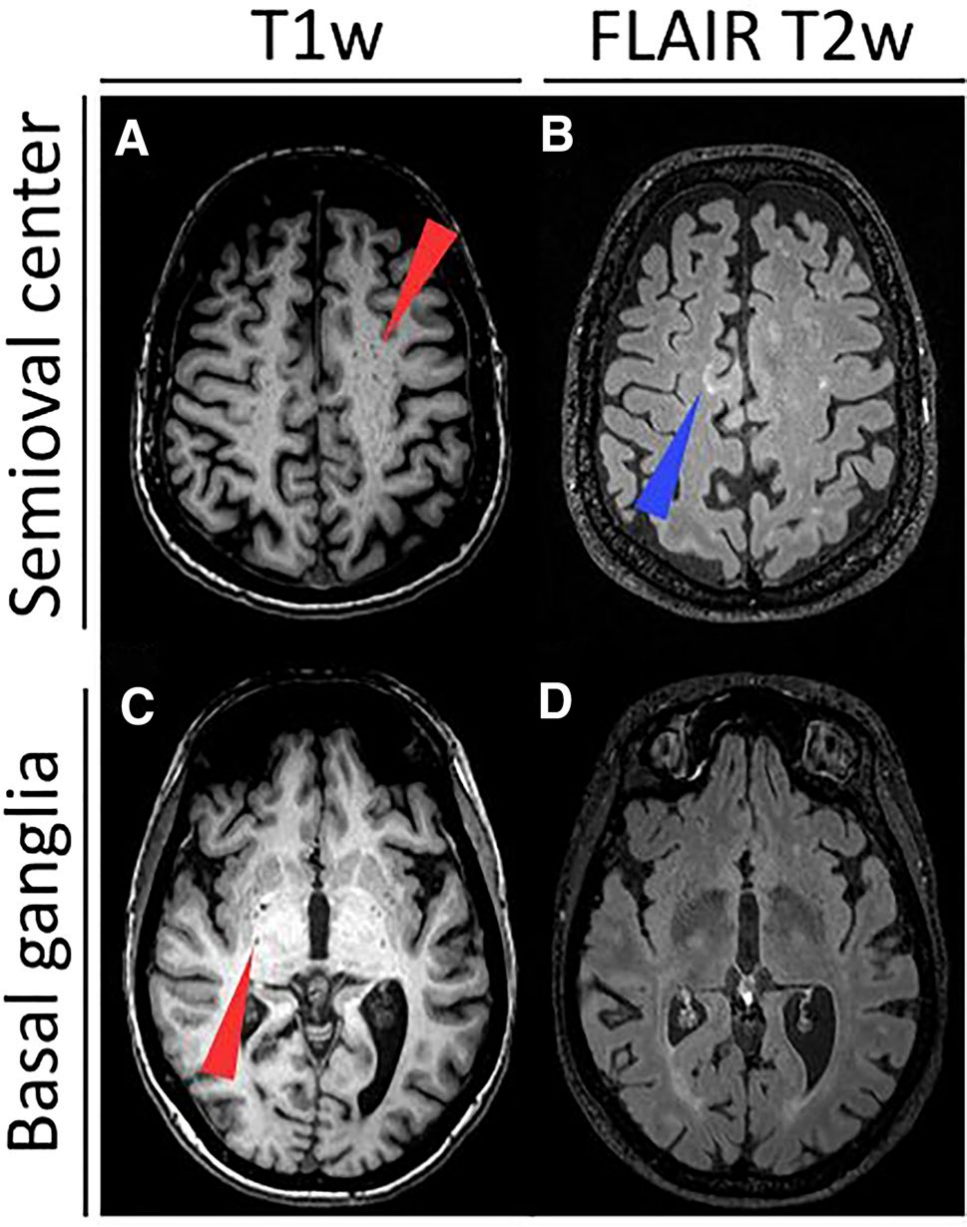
Enlarged perivascular spaces (EPVS) are readily detectable on T1- and fluid-attenuated inversion recovery (FLAIR) T2-weighted (T1w, T2w) magnetic resonance imaging (MRI) as cerebrospinal fluid-isointense punctuate structures (red arrowheads) in the semioval center (a and b) and in the basal ganglia (c and d). In contrast, multiple sclerosis lesions appear hyperintense on FLAIR T2-weighted images (blue arrowhead in b)
